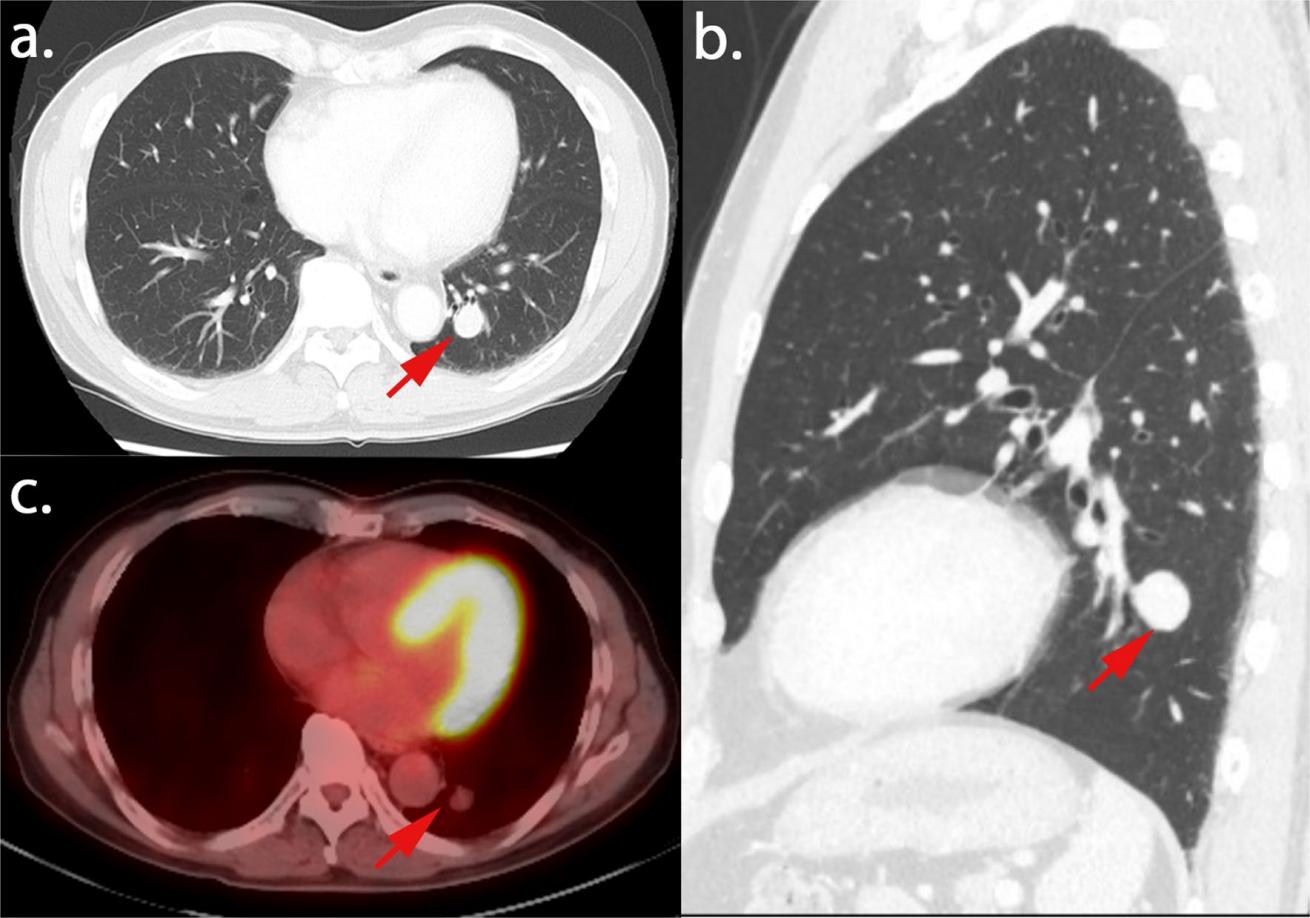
a, b Chest computed tomography findings. The nodule of the left lower lobe increased in size, and it was near the segmental bronchus. c
